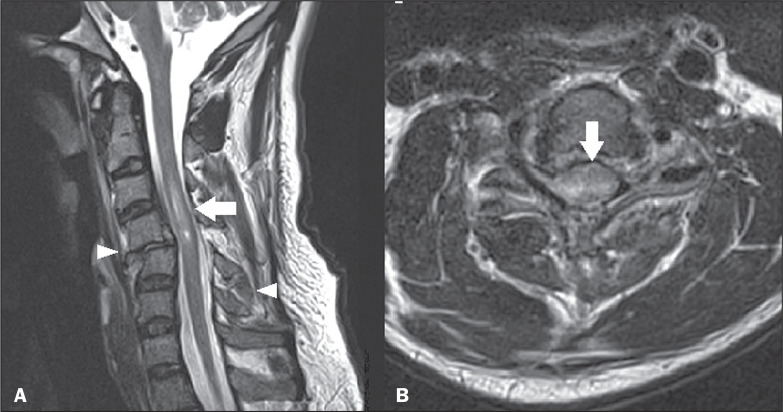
Cervical spine trauma. Sagittal and axial T2-weighted MRI sequences (A
